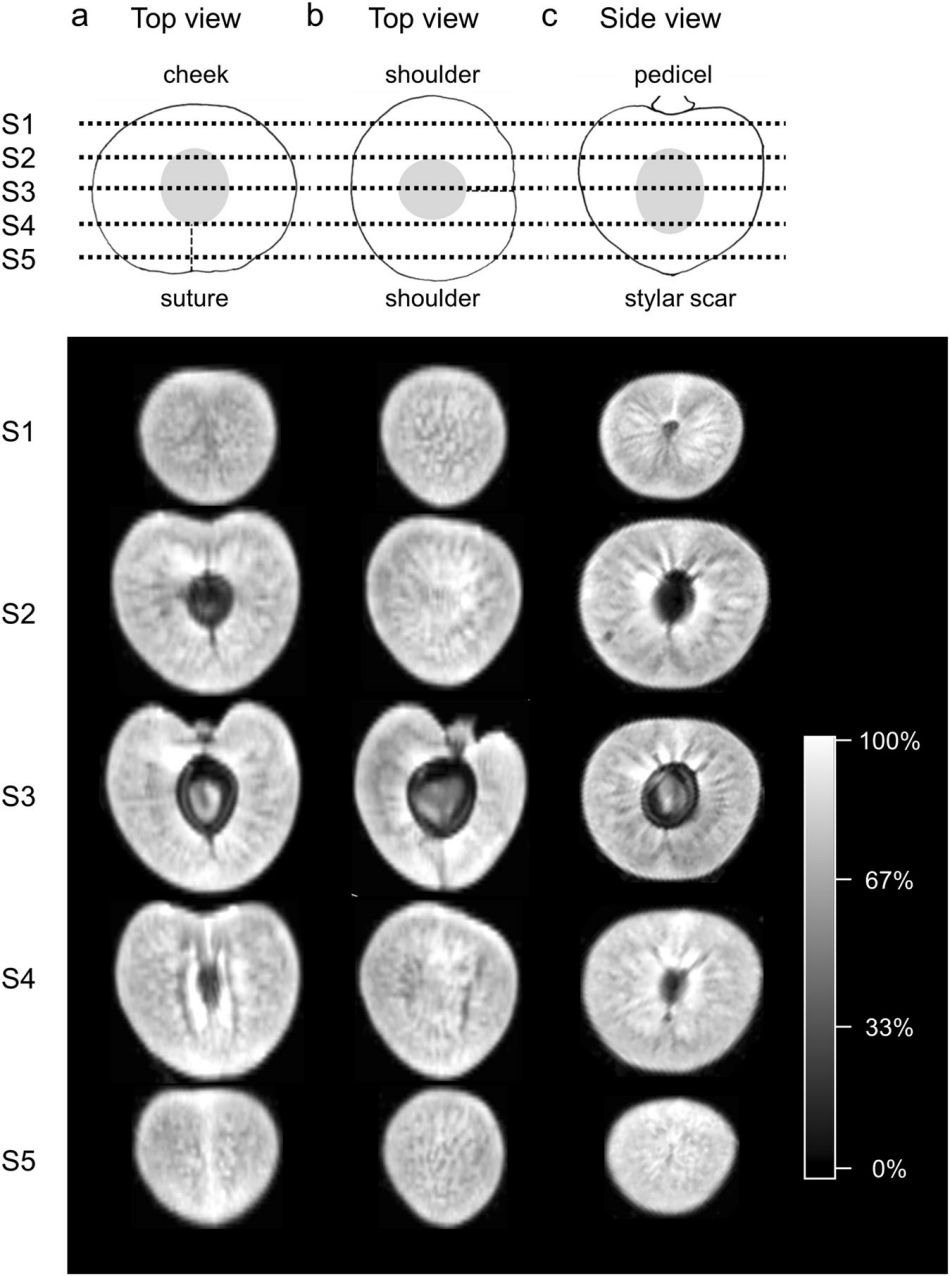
Water partitioning in a mature sweet cherry as determined by magnetic resonance imaging. Columns (a–c) represent different planes of virtual sequential sections S1 to S5 through the fruit as indicated by the dashed lines. Longitudinal sections in the plane of the two shoulders (column a), in the plane of cheek and suture (column b), and latitudinal (equatorial) sections normal to the pedicel stylar scar axis (column c)
